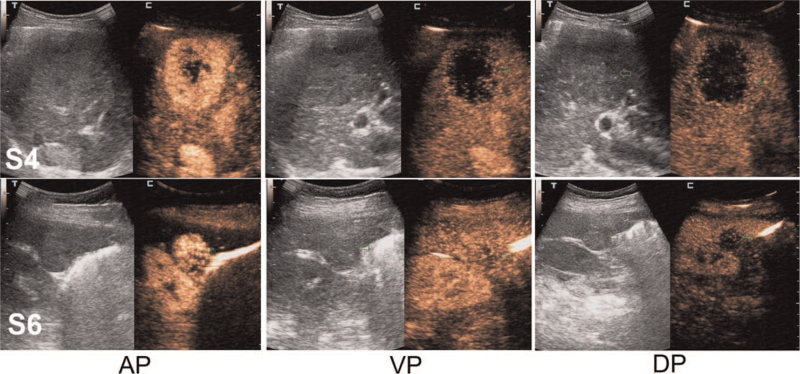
Abdominal contrast-enhanced ultrasonography (CEUS) identified 2 separate well-defined hypoechoic tumors that were heterogeneous on the inside in the segment 4 (S4) and segment 6 (S6) of the liver (green arrows; 5.0 × 4.6 cm and 2.5 × 2.1 cm in size, respectively). The S4 tumor presented high heterogeneous enhancement and the S6 tumor showed homogeneous enhancement in the arterial phase, The both tumors showed low enhancement in the portal venous phase and delayed phase. AP = arterial phase, DP = delayed phase, VP = portal venous phase.
